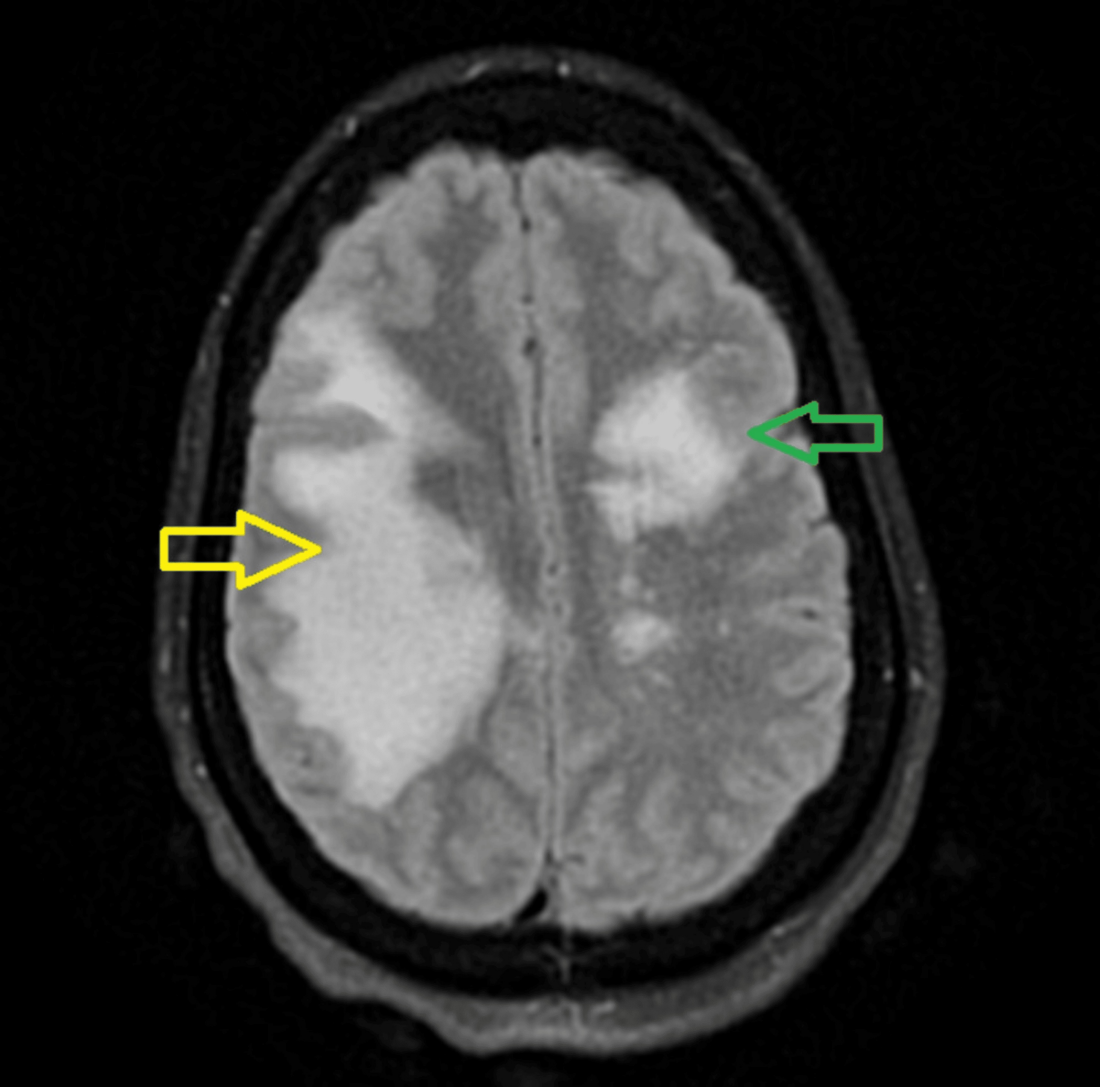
New MRI brain with and without contrast – Axial T2 FLAIR FLAIR: Fluid-Attenuated Inversion Recovery. The yellow arrow indicates a marked increase in the size and extent of the hyperintense lesion of the right frontal and parietal lobes. The green arrow indicates an interval development of a similar, moderate-sized hyperintense lesion within the corresponding white matter of the left frontal lobe.
